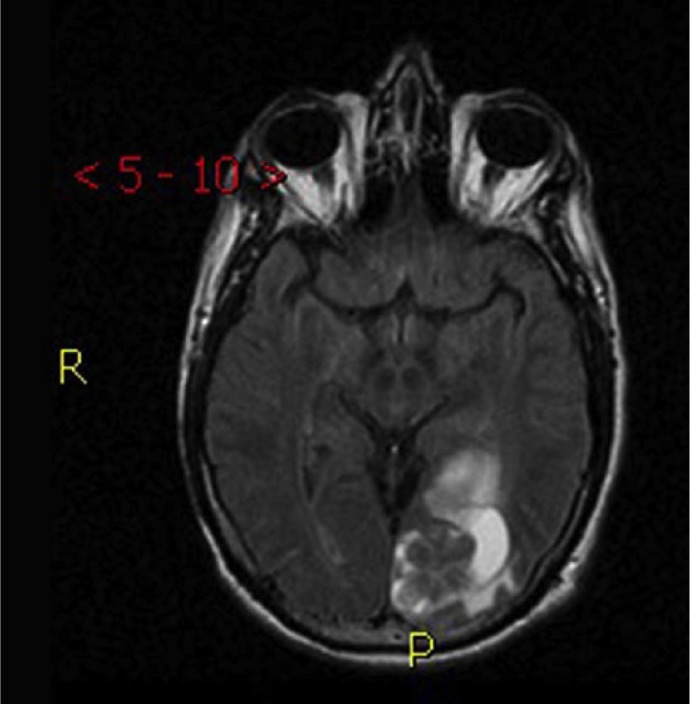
Magnetic resonance imaging scan shows the dramatic signal attenuation in the region of a large metastatic mass within the cranial vault coupled with resultant vasogenic edema.
